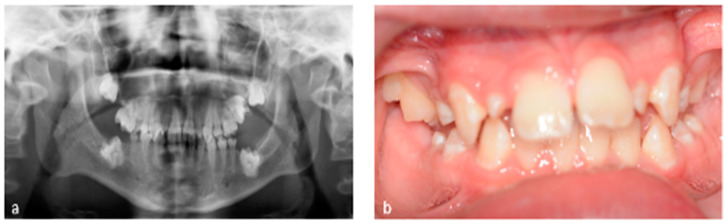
(a) Orthopantomogram showing the permanent dentition with multiple aplasia, microdontia, short and tapered roots, as well as impacted teeth and changes in condylar form in a 13-year-old patient. Stem cell transplant (for Hurlers syndrome) was performed at 2 years of age. (b) Clinical picture of this patient.
